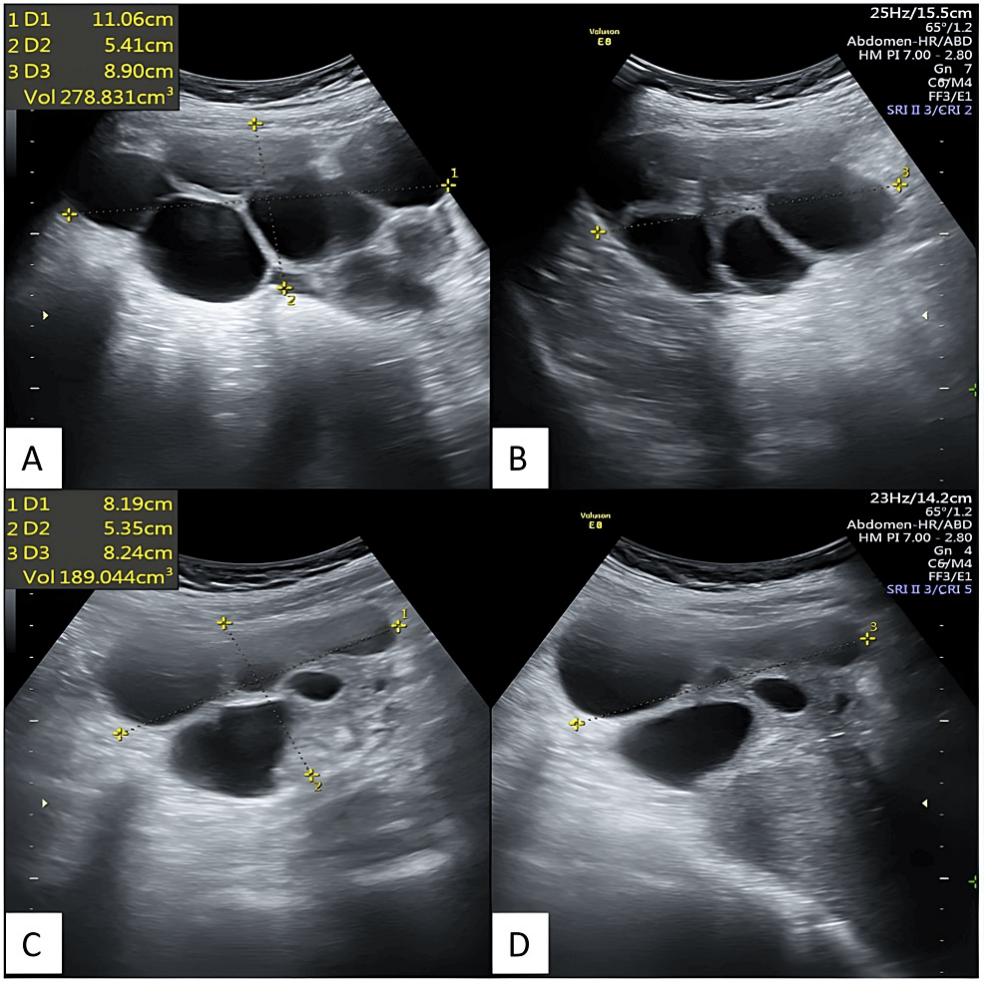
Transabdominal ultrasonography images depict enlarged bilateral ovaries with multiple cysts Transabdominal ultrasonography depicts an enlarged right ovary in axial (A) and sagittal (B) sections, and an enlarged left ovary in axial (C) and sagittal (D) sections, both exhibiting multiple cysts.
